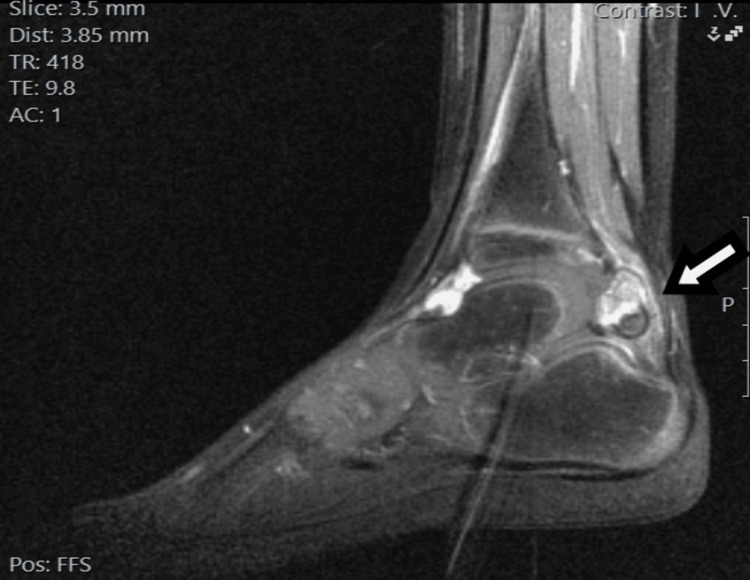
MRI scan of the right ankle T1C sequence. T1C sequence in the sagittal plane after the intravenous administration of paramagnetic agent shows inhomogeneous enhancement of the lesion.
